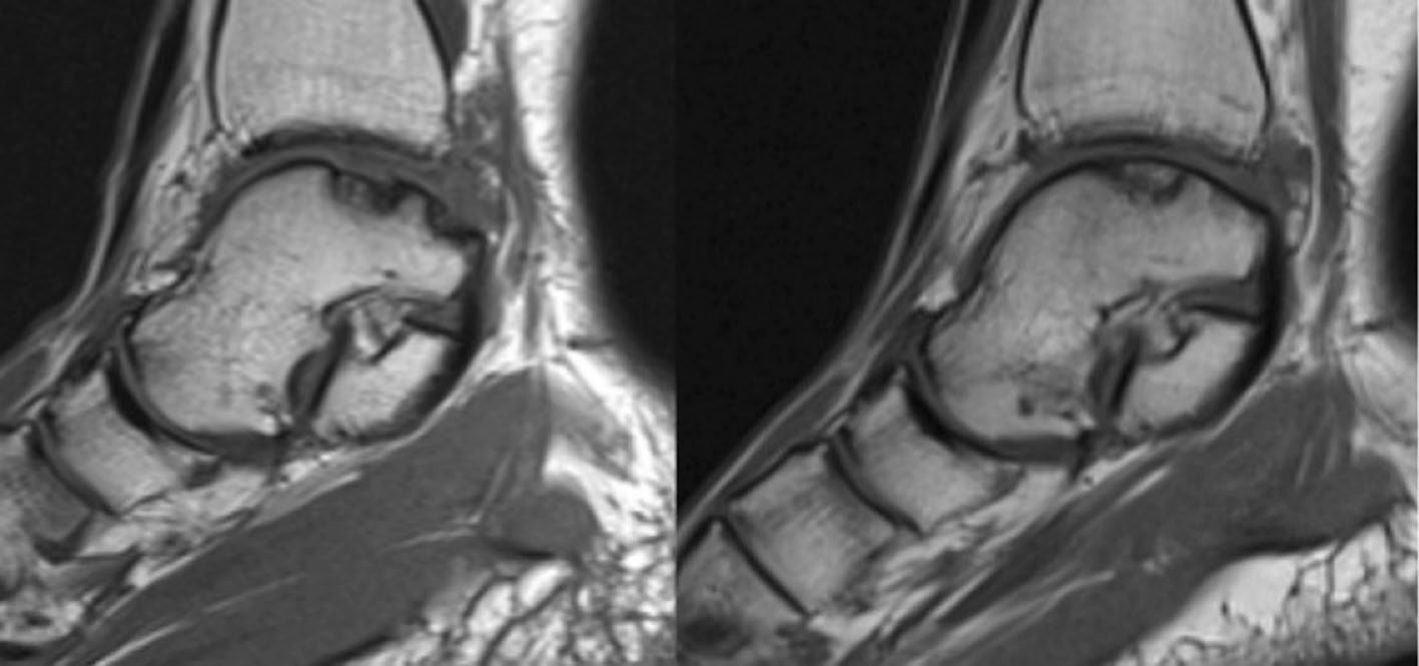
MRI comparing a OCLTs pre-operative and at 24 months follow-up. Sagittal view
